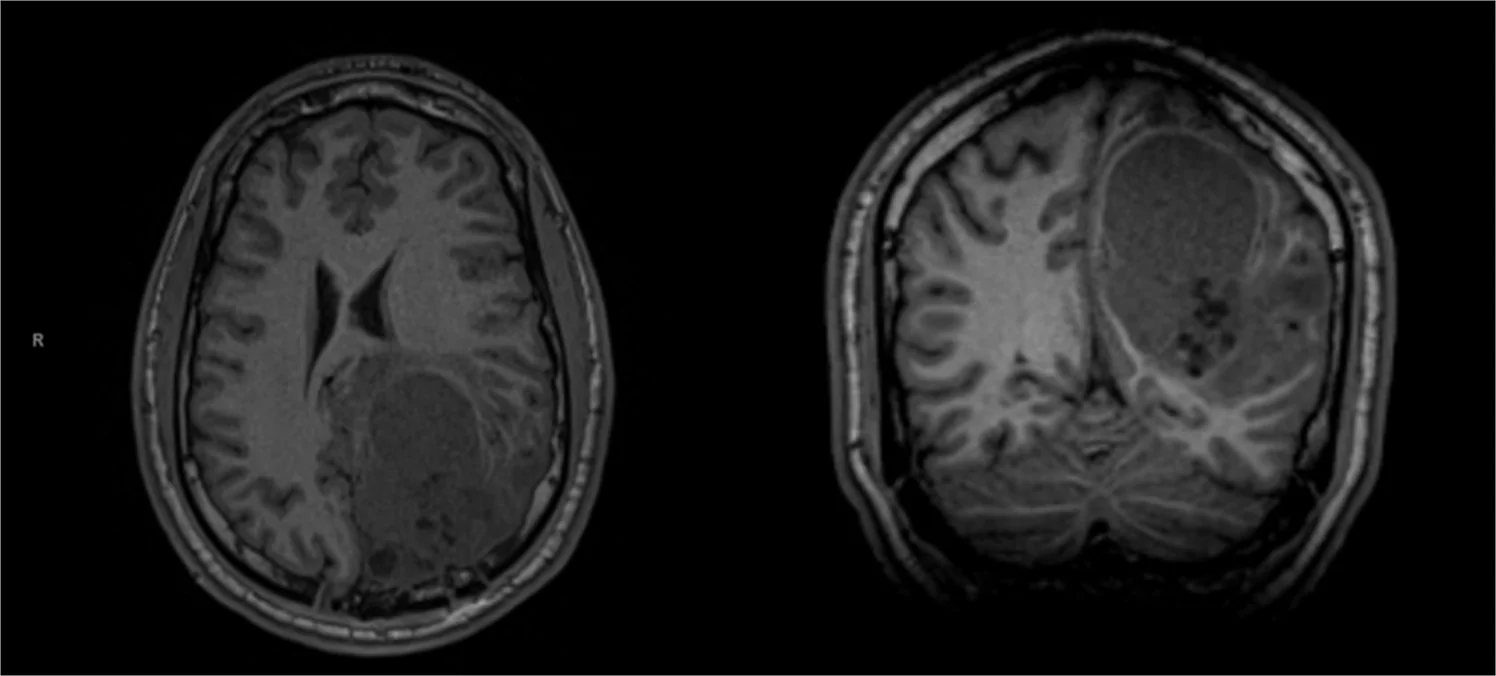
MRI brain T1 post-contrast sequence (with poor timing of contrast administration) revealing recurrence of tumor, demonstrated as a nodulocystic lesion in the left parietal lobe
